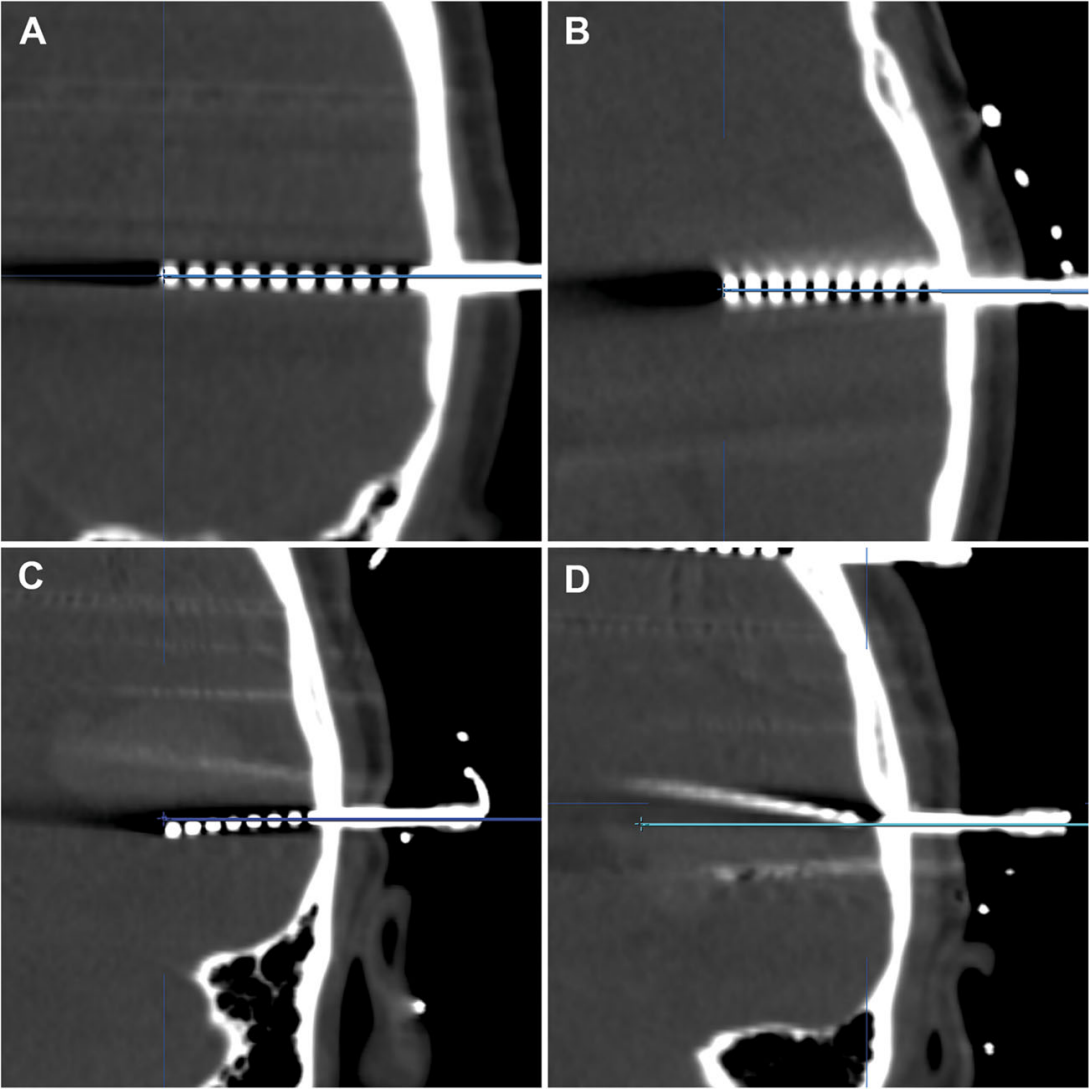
Three cases of in vivo application accuracy measurements on postoperative CT scans. The planned trajectories are shown as solid lines. For visualization purposes, the CT bone window setting was used (−200 to 800 HU). A: Coronal and (B) axial reconstructions of the same electrode, showing optimal positioning of the implanted electrode in comparison with the planned trajectory. Target point localization error (TPLE) was 0.83 mm for this electrode. C: Minor deviation in the coronal plane of an orthogonal electrode after insertion in the skull. The TPLE was 2.70 mm. D: Major deformation of the electrode in the coronal plane, with evidence of deviation in the other planes as well, resulting in a TPLE of 9.03 mm. TPLEs were measured in three different planes and calculated using the Euclidean distance
